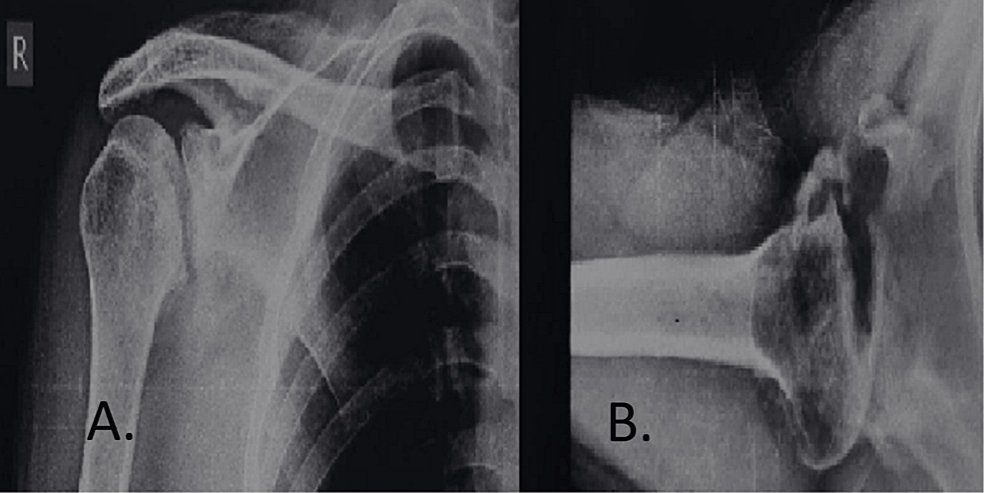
Preoperative X-ray of the patient's right shoulder depicting the anteromedial defect over the head of the humerus. (A) Anteroposterior view. (B) Axillary view.
